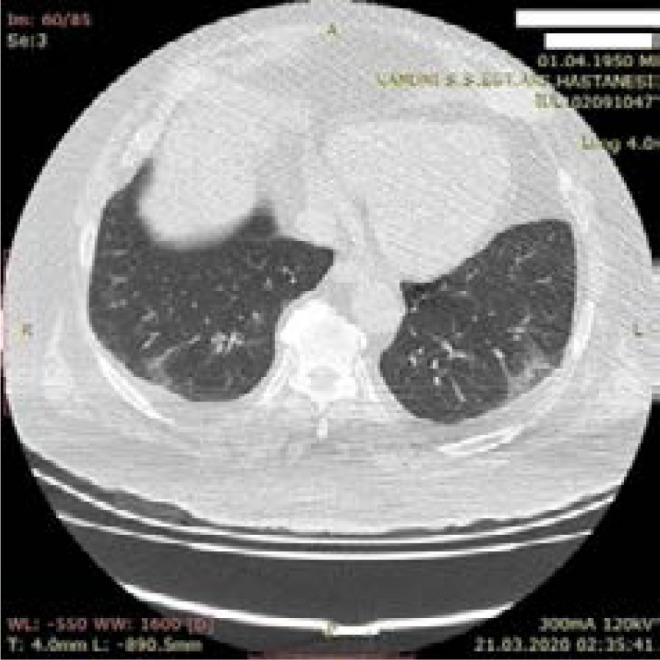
Typical mild CT finding - 60-year-old male patient -peripheral focal ground glass densities in lower lobes of both lungs
